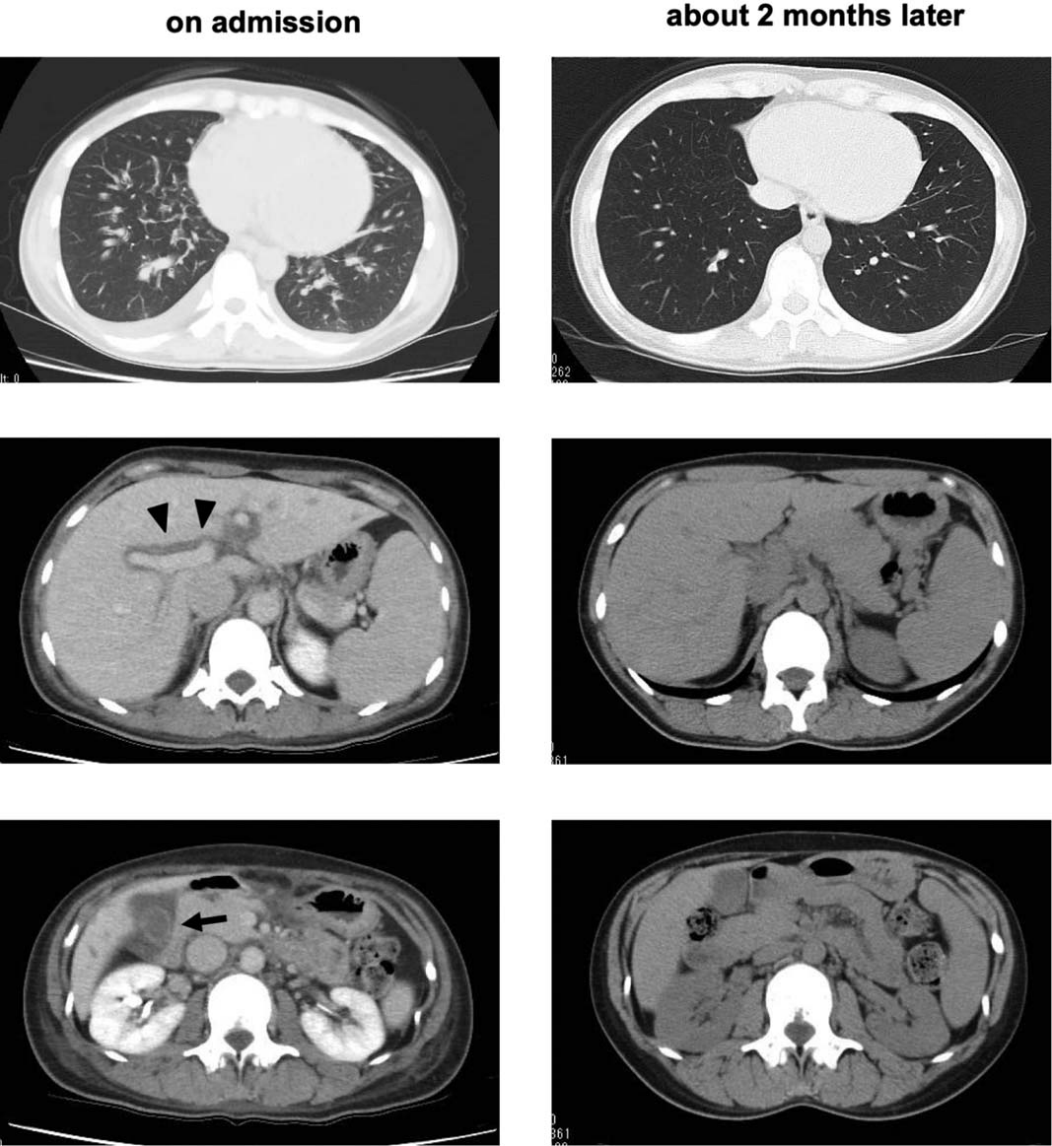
Complete disappearance of fluid retention and congestion. Enhanced chest and abdominal computed tomography (CT): Left panel, enhanced chest and abdominal CT on admission; right panel, chest and abdominal CT about 2 mo later. Enhanced chest and abdominal CT on admission revealed pleural effusion, ascites and splenomegaly. In addition, edematous wall thickening of the gallbladder (black arrow) and periportal collar sign (black arrow head) in enhanced abdominal CT. On the other hand, chest and abdominal CT about 2 mo later revealed the disappearance of edematous wall thickening of the gallbladder which was likely associated with the improvement of thyroid function.
